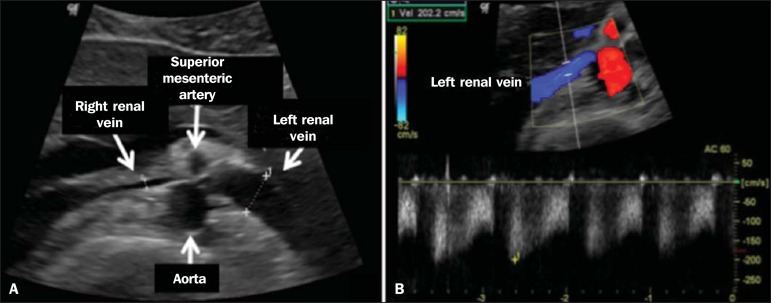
Nutcracker syndrome. A: B-mode ultrasound showing left renal
